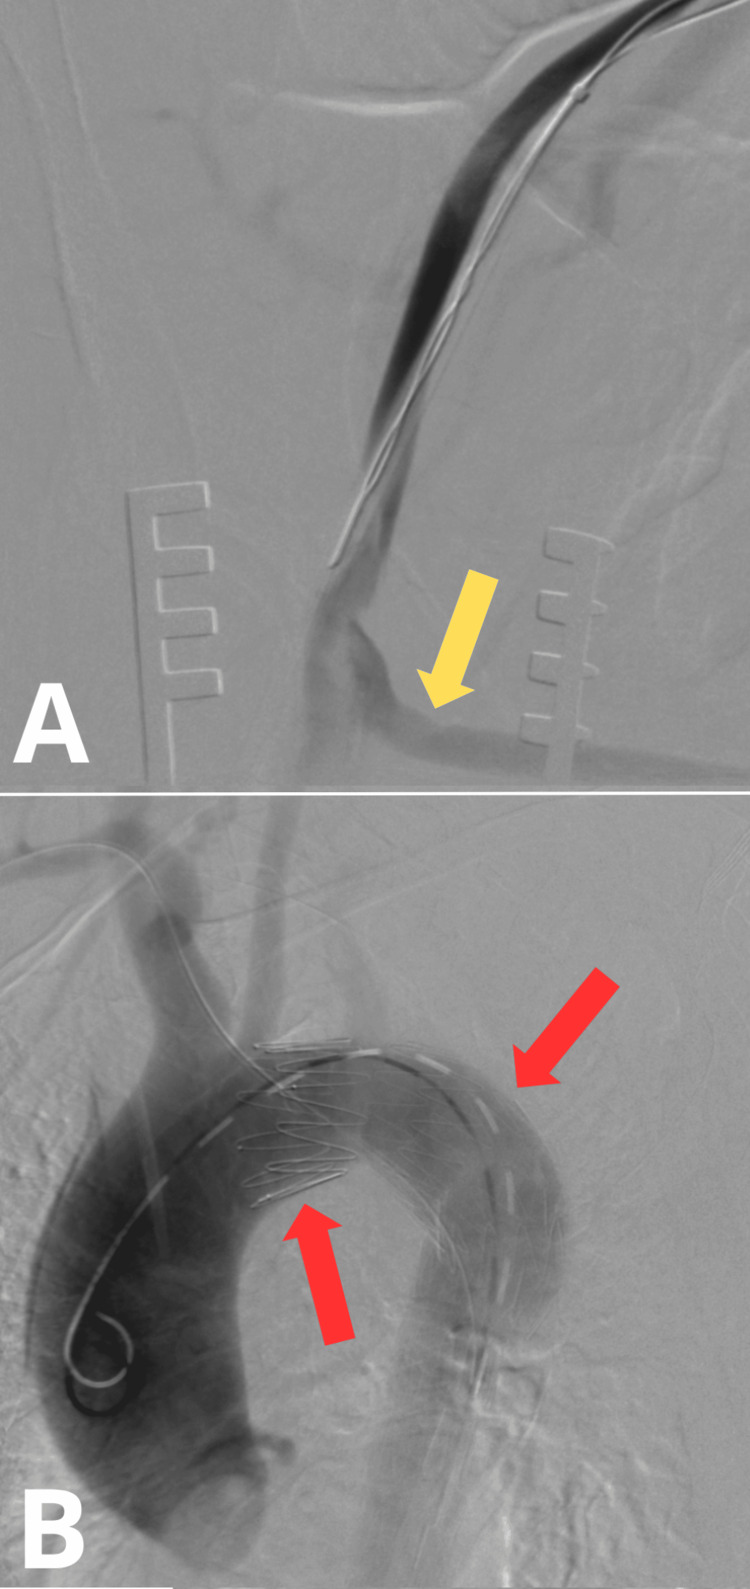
Intraoperative angio-CT Scan: (A) the View of the Patent Femoro-Femoral Bypass Graft and (B) the View of the Stent Graft in the Aortic Arch The yellow arrow indicates the left femoro-femoral bypass graft. The red arrow indicates the stent graft CT: computed tomography
